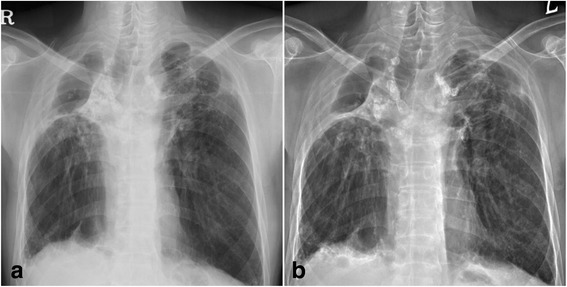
Simple chest radiographs of Case 1. a In 2011, pulmonary tuberculosis sequelae with fibrosis scarring were observed. Reticulations were observed in both lower lobes. Pleural thickening and calcification were found in the right lower lung. b In 2016, fibrocalcifications of both the upper lungs and the right lower lung were observed. Emphysematous bullae were observed in both the upper lungs. The reticular pattern and number of bullae increased as compared with 2011
